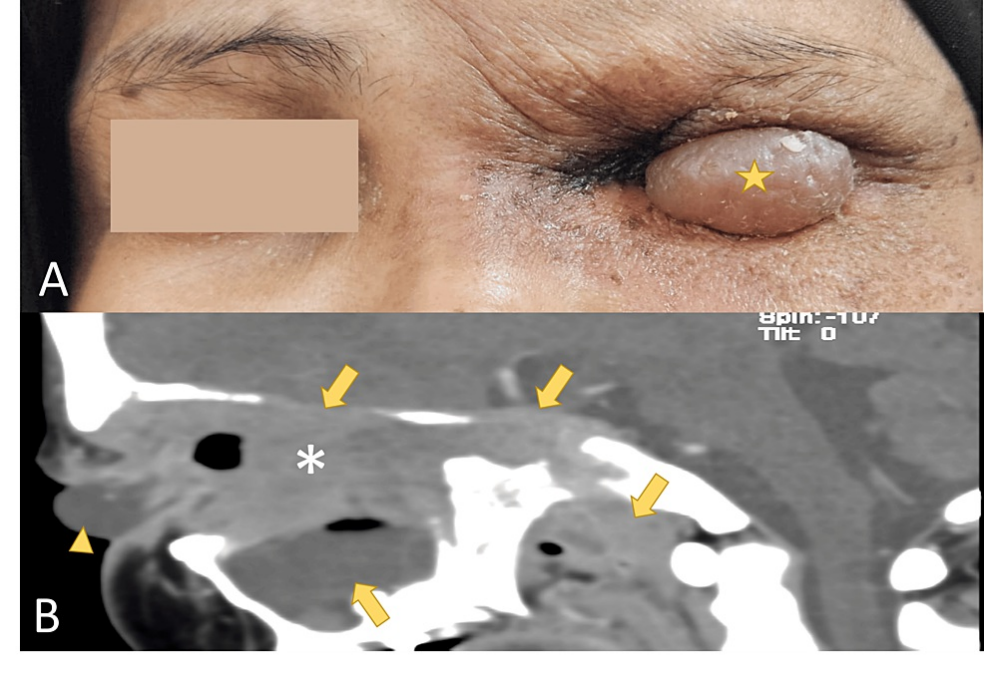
Recurrence after modified enucleation. Recurrence of the non-cutaneous squamous cell carcinoma (ncSCC) over the left eye. (A) Well-defined cystic mass that involves the upper eyelid (star). (B) The sagittal view of contrasted computed tomography orbit showed enhancing extensive tumor (asterisk) occupying orbit and invading maxillary sinus, cavernous sinus, and medial cranial fossa with bony erosion (arrow). A cystic lesion (arrowhead) was arised from the tumor anteriorly that coincides clinically.
